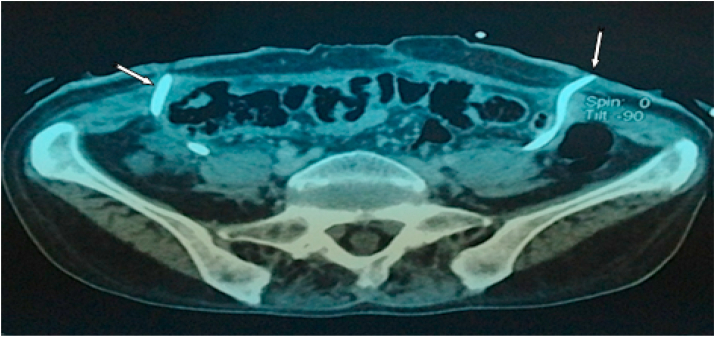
Thoraco-abdominal-pelvic CT scan showed Infiltration of pelvic fat with no detectable mass. Ureterostomies are connected to the abdominal wall.
